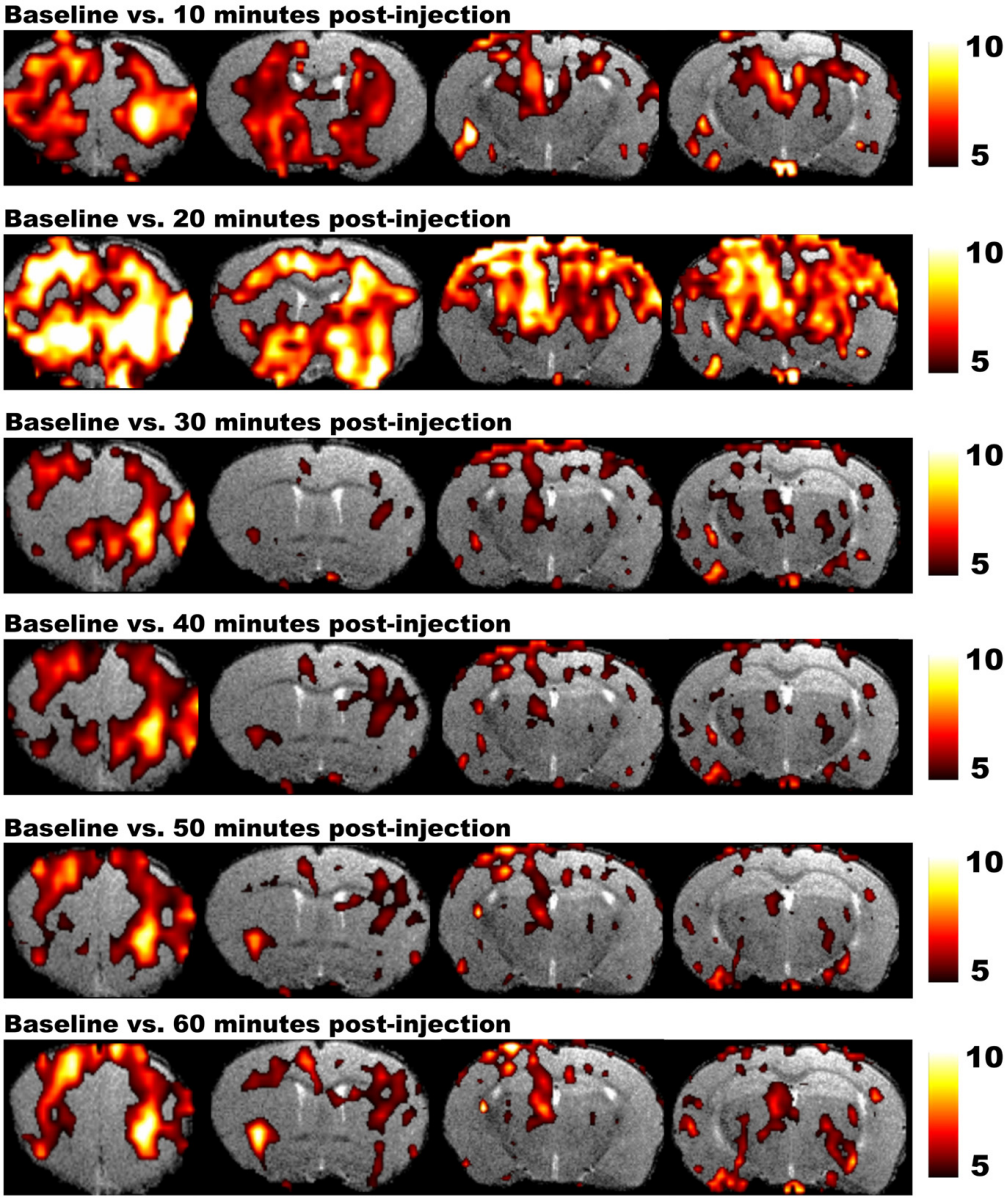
Results of phMRI with a single bolus of medetomidine. This figure shows four consecutive slices of the statistical difference maps of the BOLD signal at 10, 20, 30, 40, 50, and 60 min post-injection (medetomidine, 0.3 mg/kg, s.c.) vs. baseline. The statistical maps are shown on a T2-weighted anatomical MRI template. The color scale at the right indicates the *T*-value (i.e., the strength of the T2^∗^ signal intensity decrease induced by medetomidine vs. baseline in all conditions). Medetomidine induces T2^∗^ signal changes at 10 min post-injection vs. baseline, mainly in frontal regions and the striatum. At 20 min post-injection, the T2^∗^ signal changes increase and include additional regions such as the sensory cortex, hippocampus and thalamus. Starting from 30 min post-injection, medetomidine-induced T2^∗^ signal changes start to decrease and stabilize until 60 min post-injection (Shah et al., 2015a).
